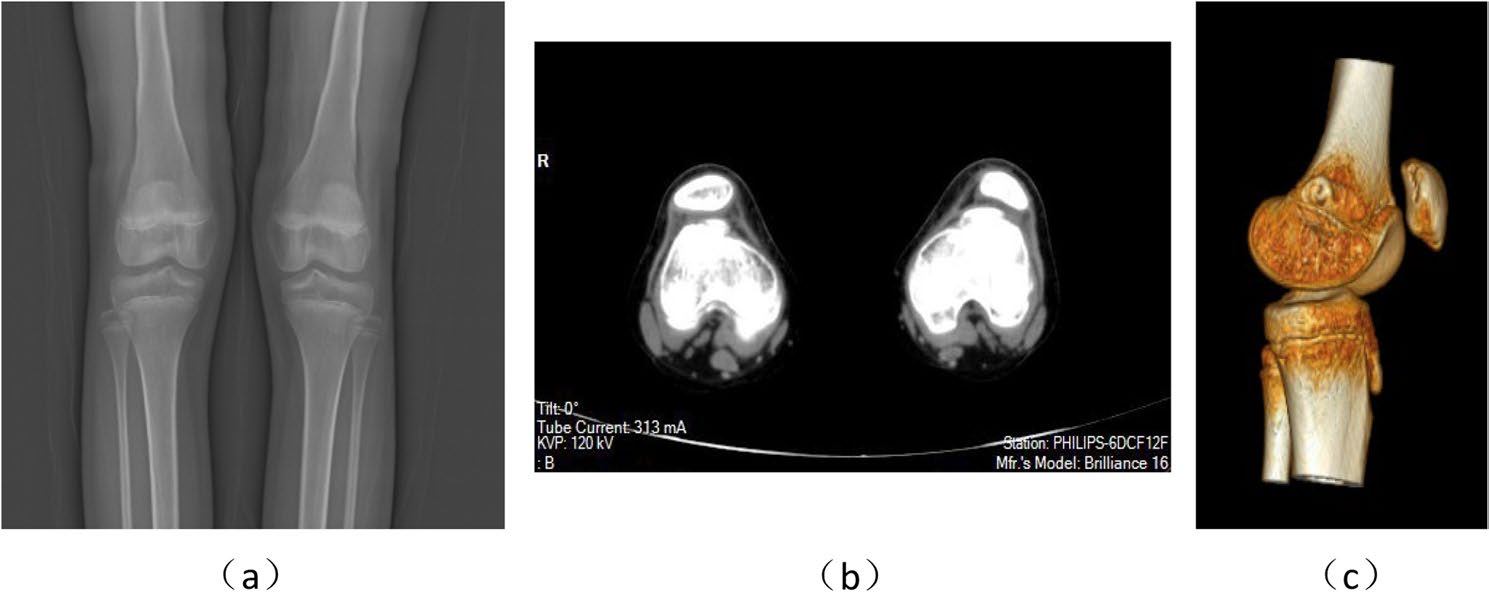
Preoperative radiograph and computed tomography (CT) images of the knee joint in case 2. a The anteroposterior radiograph of the bilateral knee joints shows the unclosed epiphysis and left subluxated patella; b The horizontal CT image of the bilateral knee joints shows Dejour type C trochlear dysplasia and that the bilateral patella is not in the trochlear groove; c The three-dimensional reconstruction of the right knee joint demonstrates patella alta
